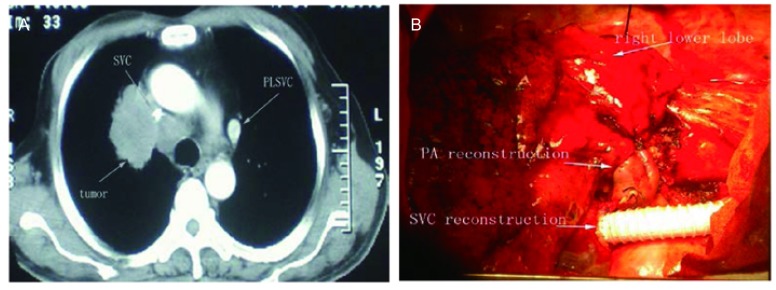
Image of lung cancer and surgery. A: CT scan revealed the tumour infiltration of SVC and the PLSVC; B: The SVC replaced by interpositon of a 12 mm ringed PTFE graft and the sleeve reconstruction of bronchus and PA. CT: computed tomography; SVC: superior vena cava; PLSVC: persistent left SVC.
